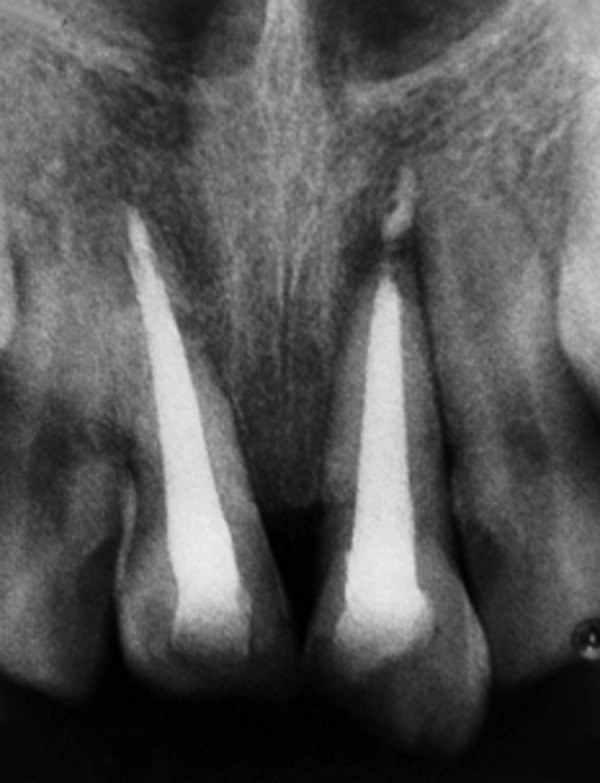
Postoperative intraoral periapical radiograph (case 2)
